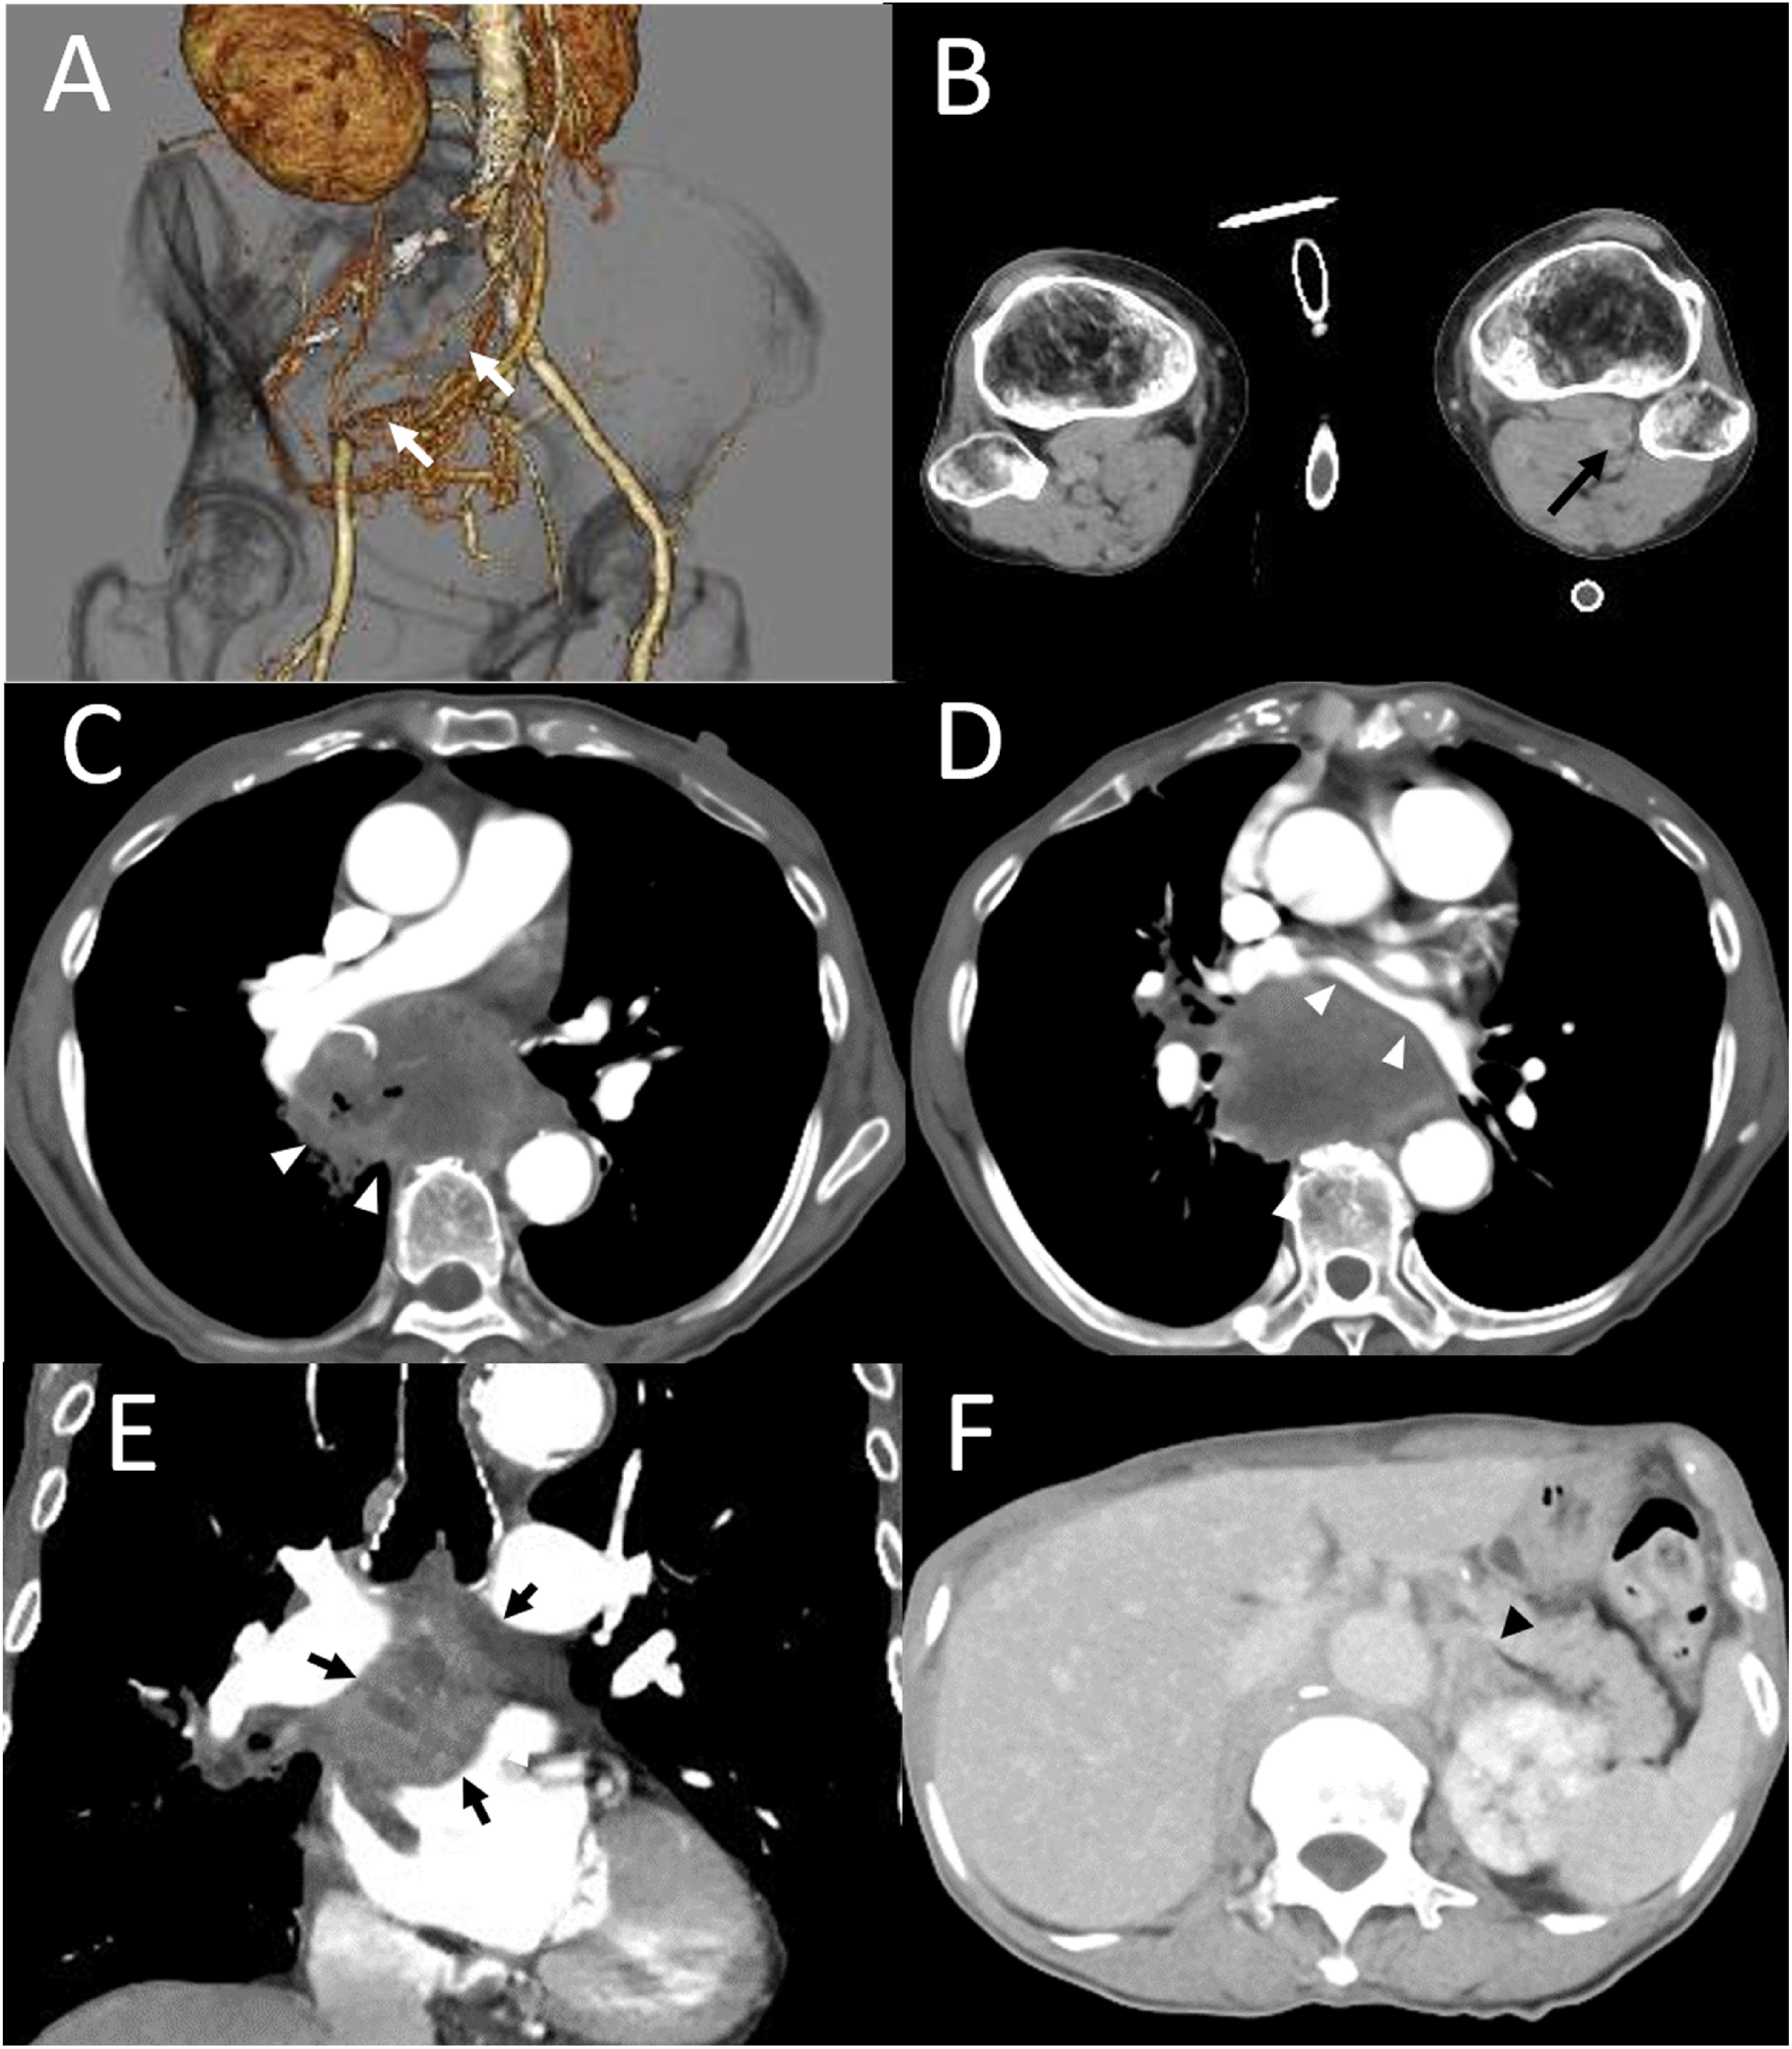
Fig. 1 Images of the computed tomografic scan. (A) Arrows indicate the occluded right common and external iliac arteries. The left iliac artery was patent. (B) The deep venous thrombus was observed in the left popliteal vein, as indicated by the arrow. (C) The tumor occupied and obstructed the right middle and lower lobar bronchi, as indicated by the arrowheads. (D) The tumor pressed the left atrium (LA) and left superior pulmonary vein, as pointed out by the arrowheads. (E) The arrows point to the mass in the LA. There was no clear distinction between the tumor invading the right main bronchus and the mass formed in the LA. (F) The left adrenal gland was swollen with a mass, as indicated by the arrowhead.
